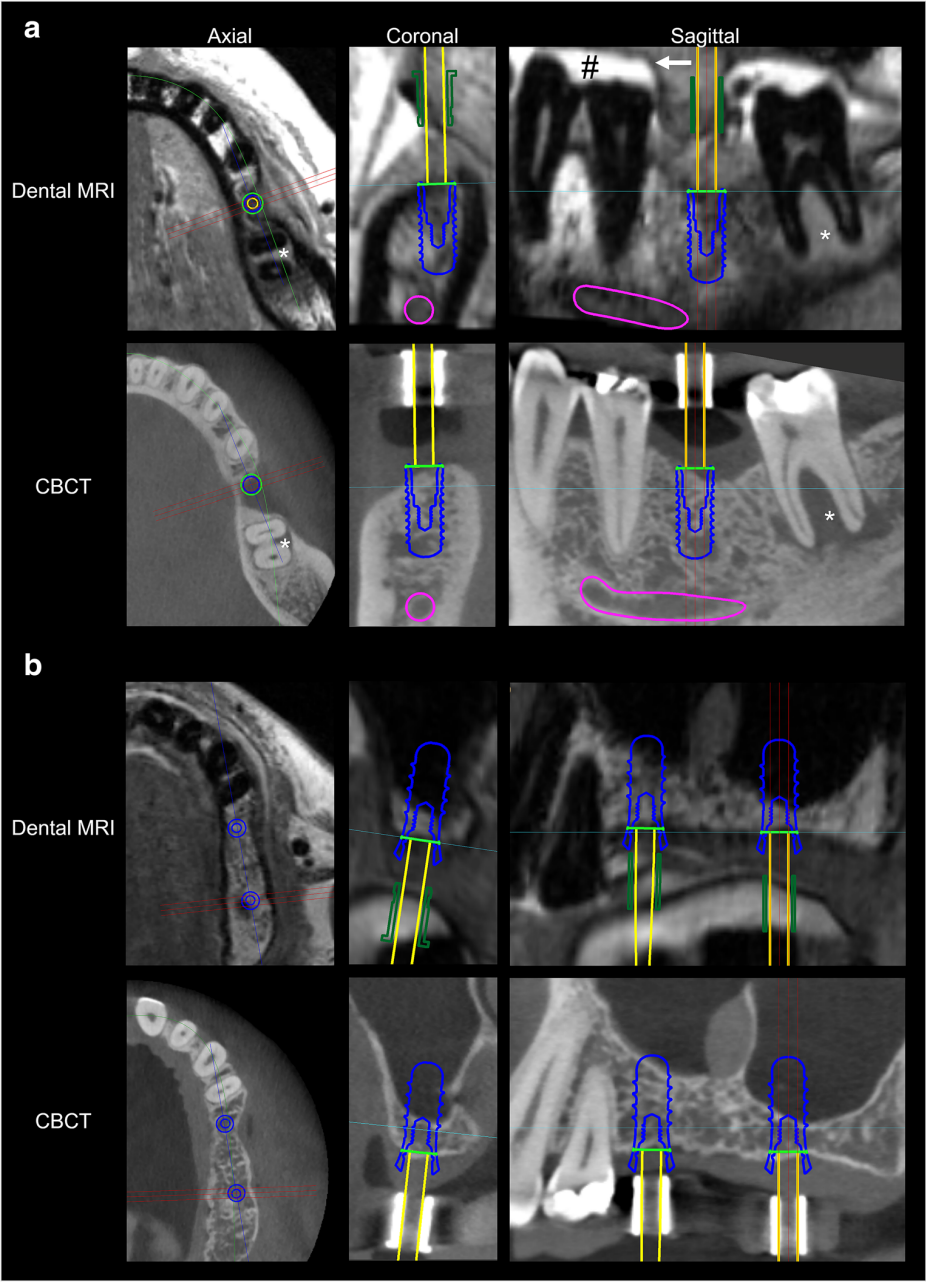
Direct comparison of two examples of implant planning in dental MRI and CBCT. In example a, implant insertion was planned in region 36. Based on dental MRI and CBCT images, no bone augmentation was necessary. In addition, a large combined periodontal-endodontic lesion can be seen in region 37 in both imaging modalities. Note the good delineation of the tooth surfaces against the bright toothpaste (#) in the splint (arrow). In example b, implant insertion was planned in regions 25 and 27 (coronal images from region 27). For both imaging modalities, it was decided that sinus lift augmentation was necessary in both regions
